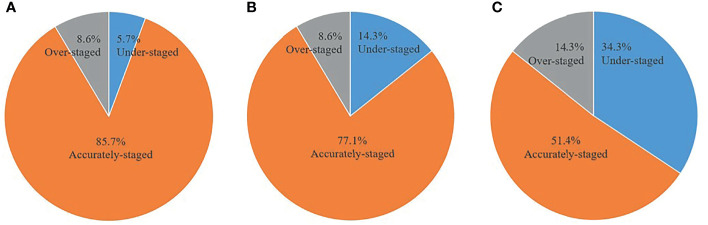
Comparison of accuracy between PET/MRI, MRI, and CECT in primary tumor assessment. (A) PET/MRI. (B) MRI. (C) CECT.
